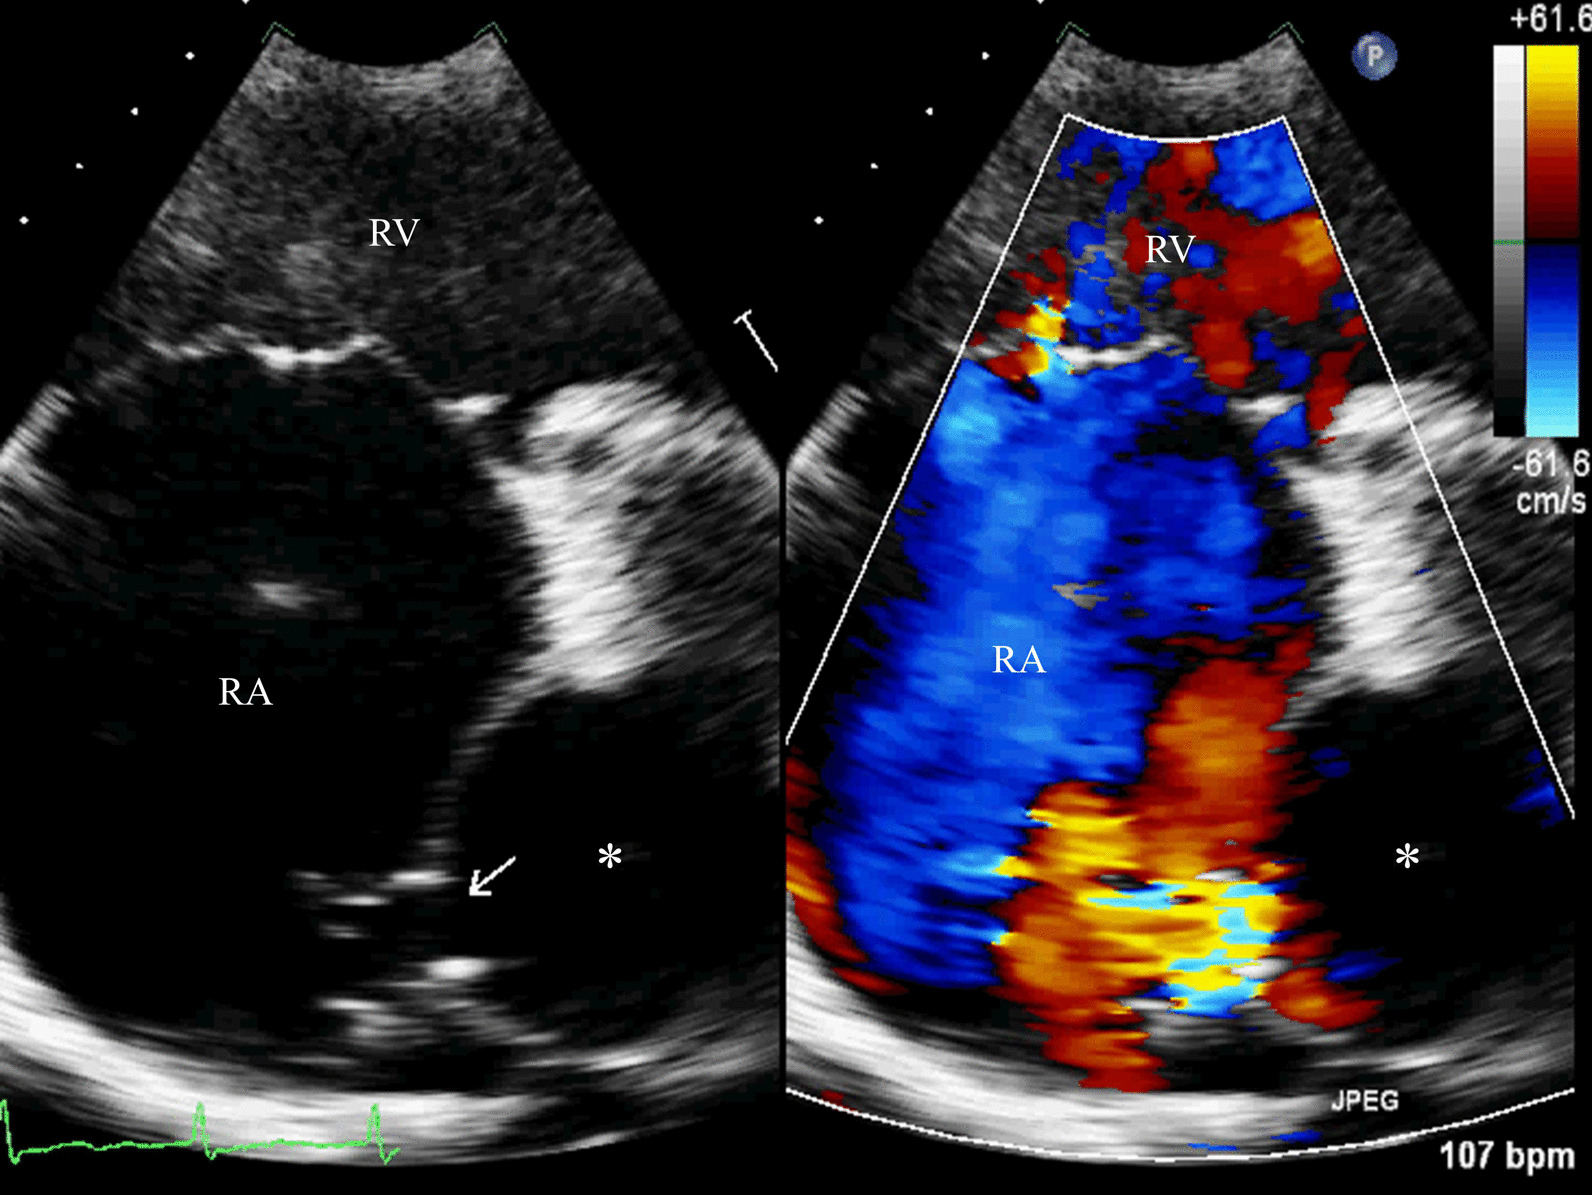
Transthoracic echocardiography revealed the LCA terminated in right atrium (arrow), proximal part of LCA was enlarged and the aneurysm (asterisk) was measured 56 × 45 mm (RV: Right ventricle; RA: Right atrium)
